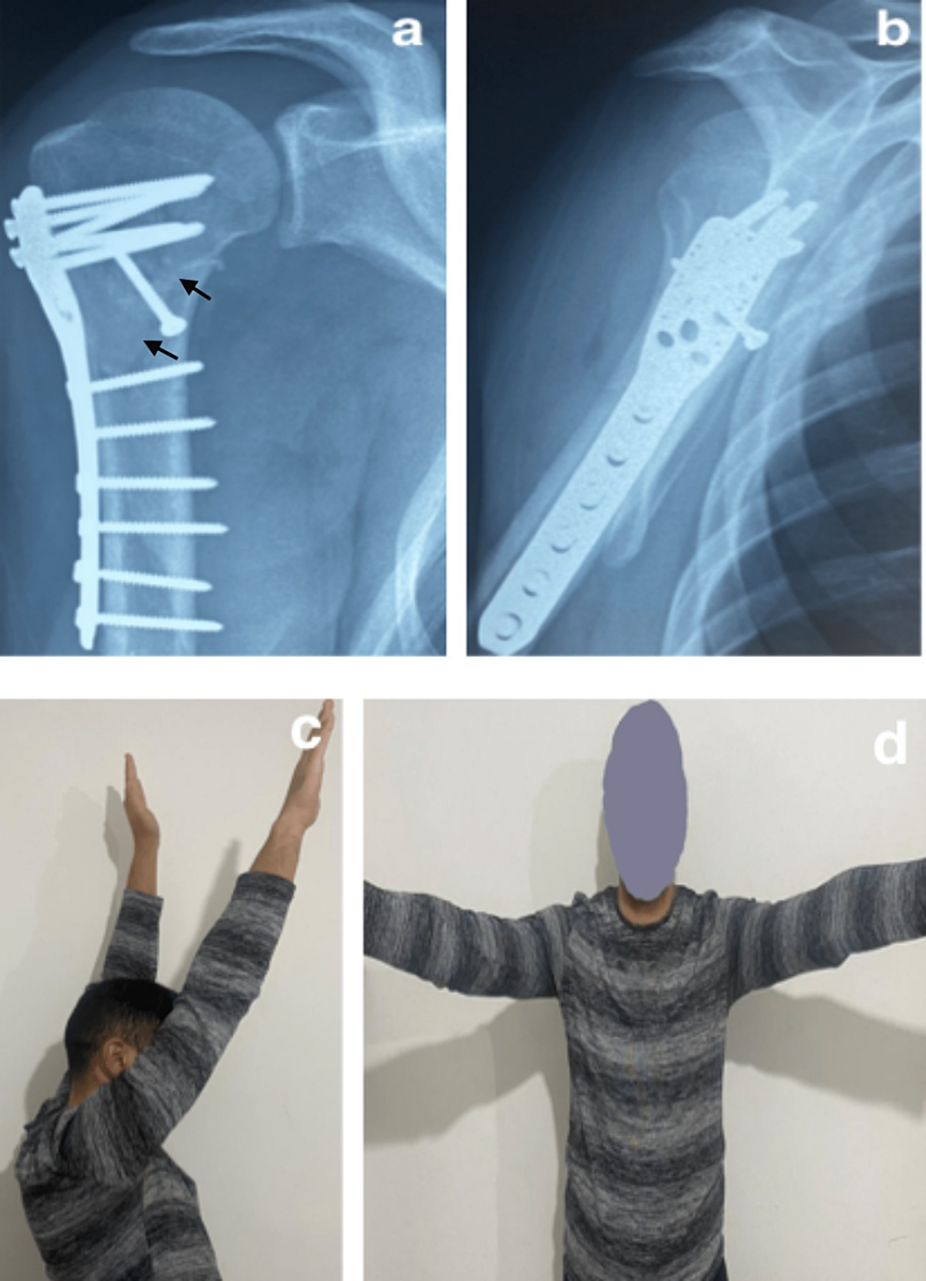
(a, b) Radiographs at six weeks showing consolidation of the posterior fracture-dislocation (black arrow), (c, d) with satisfactory shoulder function at six months.
